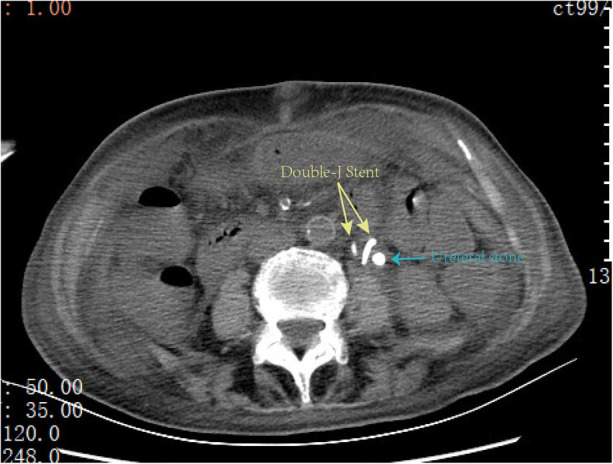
Abdominal CT image on the 1st postoperative day.
